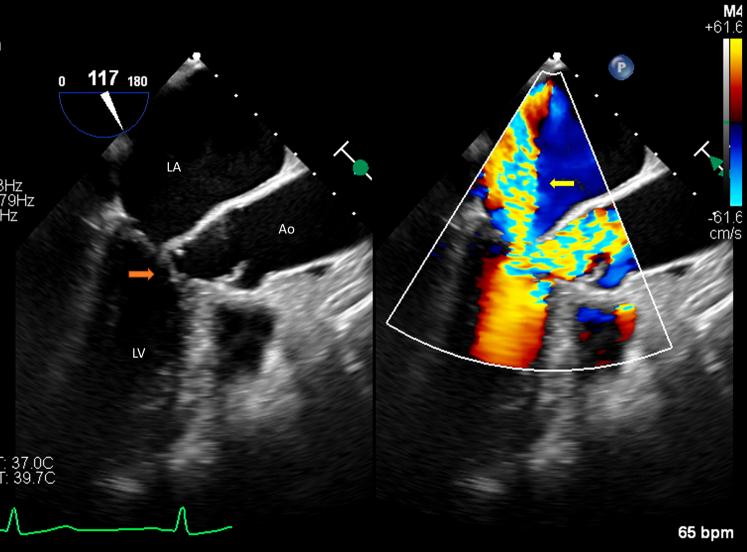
Transesophageal Echocardiography of the Mitral Valve Long-axis midesophageal view of the mitral valve at ∼120° demonstrating systolic anterior motion (red arrow) with resultant moderate to severe posteriorly directed mitral regurgitation on color Doppler (yellow arrow).
